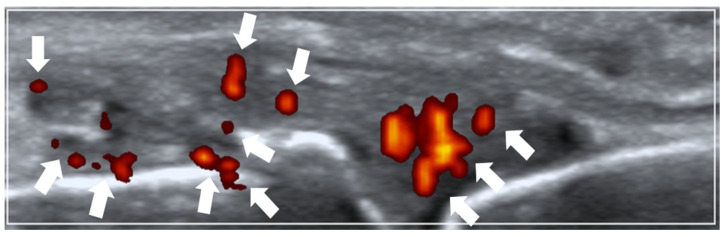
Ultrasound power Doppler (PD) joint inflammation. Example of a sonogram (longitudinal view) showing grade 2 PD joint inflammation at the metacarpophalangeal joint (arrows pointing towards PD vascularity).
